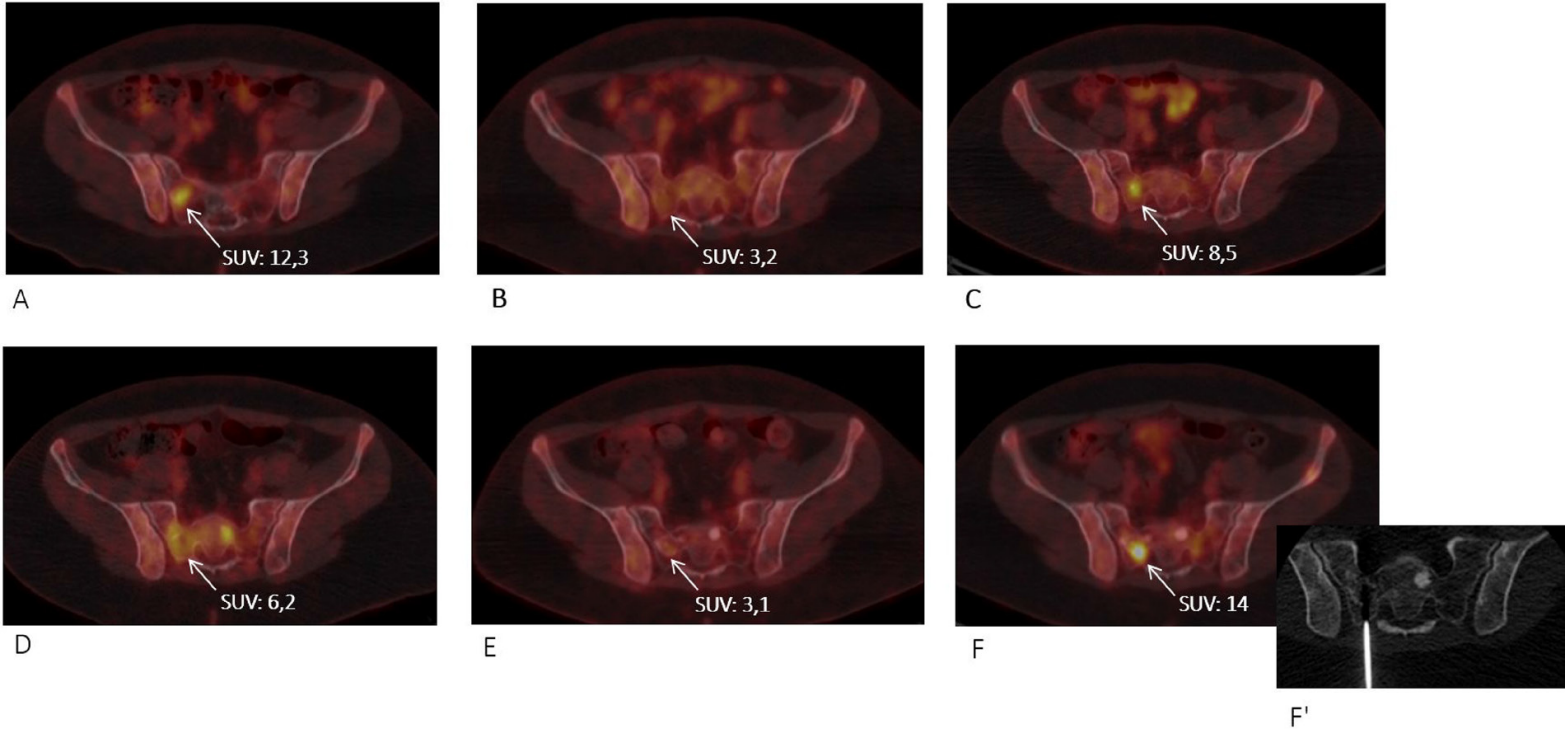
Axial F^18^–fluorodeoxyglucose PET/CT images at the pelvic level showing the right sacral alae lesion (arrow) chosen as a target for molecular analyses after progression to temozolomide (A) Before vismodegib monotherapy, (B) partial response to vismodegib, (C) disease progression under vismodegib, (D) further lesion size progression under itraconazole, (E) partial response to temozolomide, and (F) progression under temozolomide when the CT scan-guided biopsy was performed (F’).
